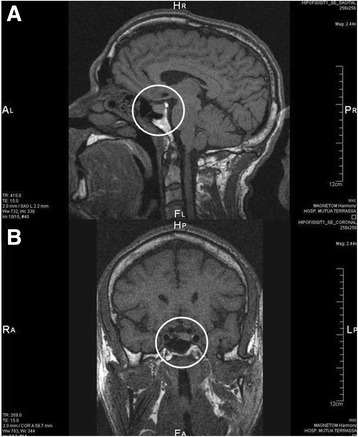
MRI study of the pituitary gland with IV contrast medium. Tomography in a) sagittal and b) coronal planes. Left intrasellar lesion measuring 11.5 × 9.5 mm touching the cavernous sinus wall. Note the normal glandular tissue on the right, the deformity of the floor of the sella, and the central position of the pituitary stalk
